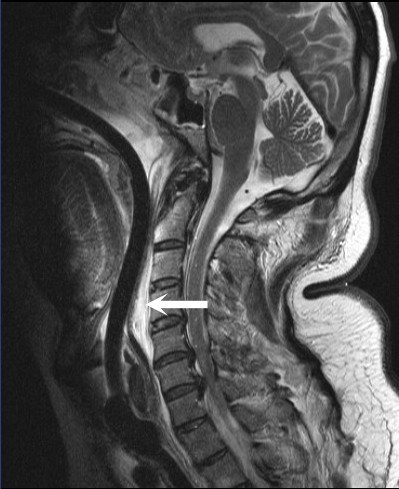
After surgical decompression, hematoma size is demonstrably reduced (arrow) in this sagittal MRI.
